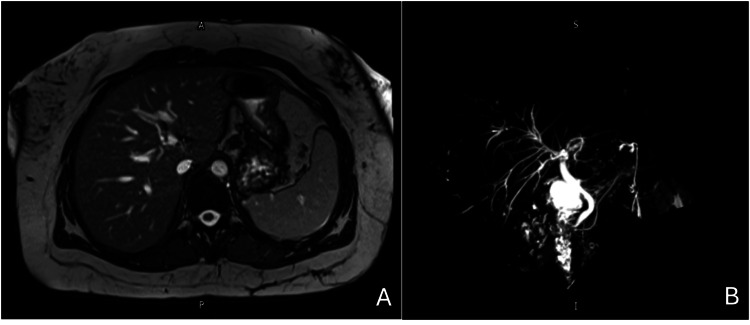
T2-weighted magnetic resonance image of the liver (A). Magnetic resonance cholangiopancreatography (MRCP) showing normal biliary tree (B).
